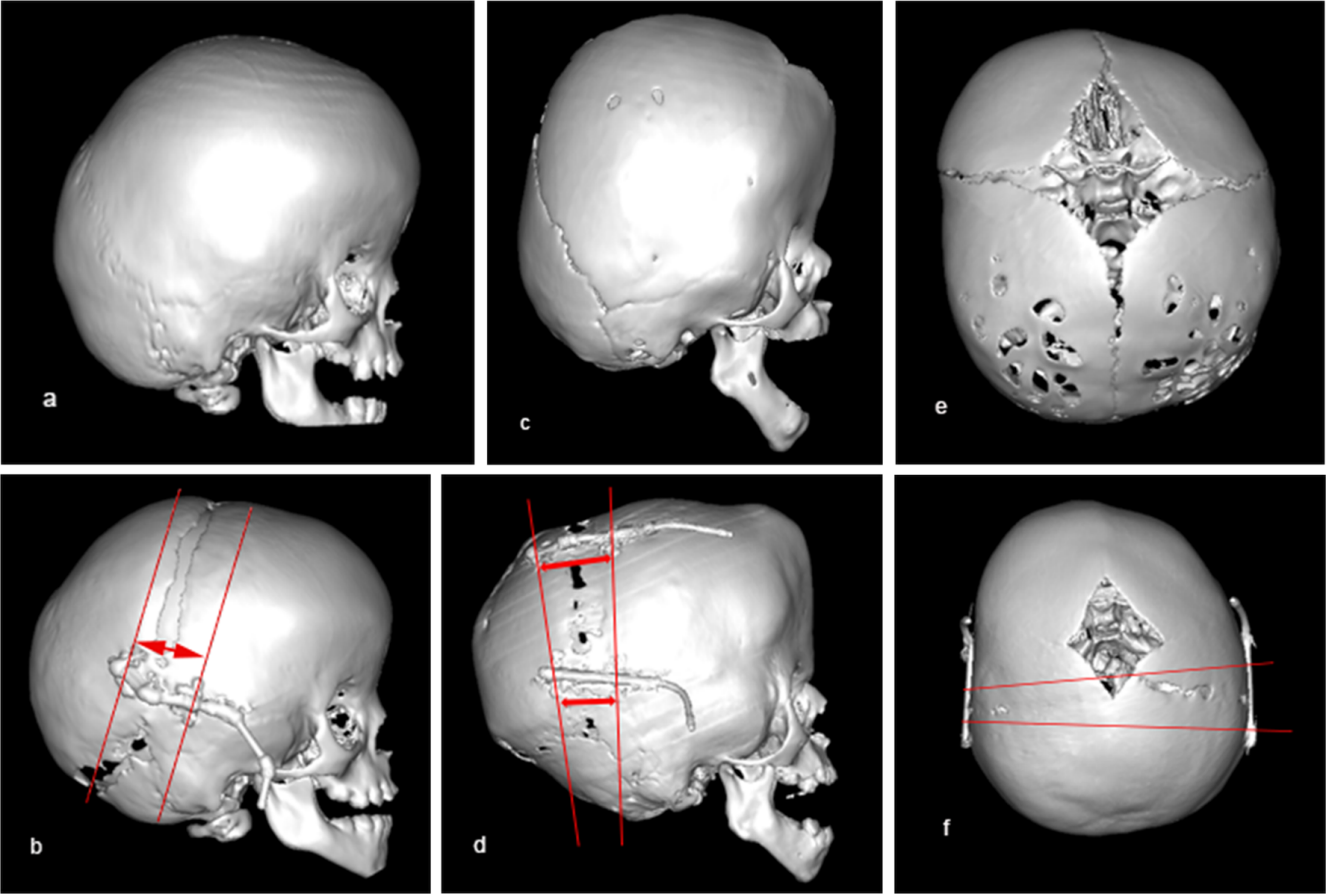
3D reconstructions of the skull CT images presenting different distraction options. Conventional PCVD with posterior shifting of the bone: a preoperative image and b image after the treatment was completed; PCVD with rotation of the bone flap along the lower occipital osteotomy line: c preoperative image and d image after the treatment was completed; asymmetrical PCVD in patient with bi-coronal and right lambdoid synostosis: e preoperative image and f image after the treatment was completed
